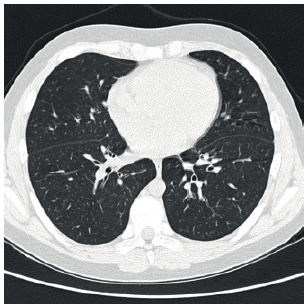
HRCT scan of the chest showing mild, slightly heterogeneously distributed
